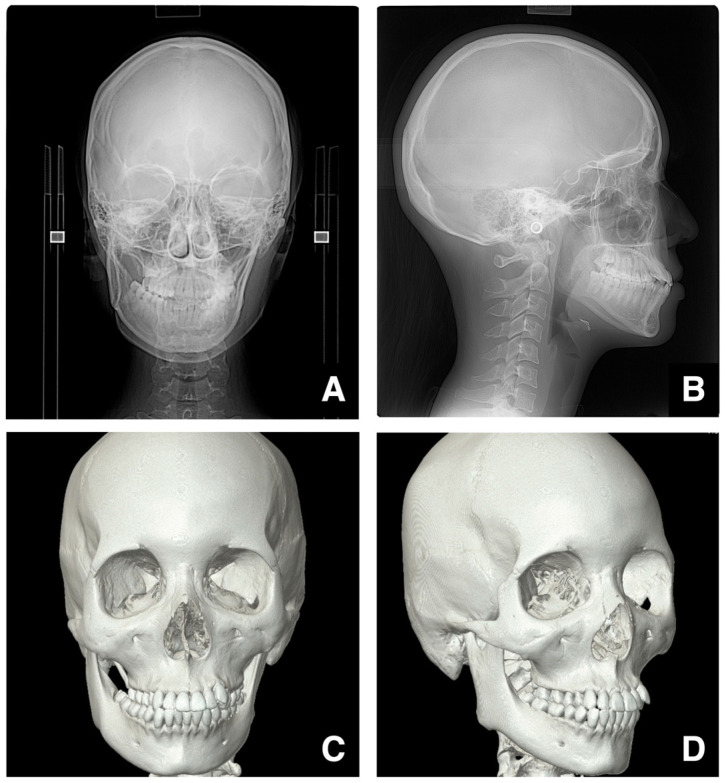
Three-dimensional reconstructed images using CT data and cephalometric radiographs in skeletal mandibular prognathism with facial asymmetry. (A) Frontal cephalometric radiograph of a patient with skeletal mandibular prognathism and facial asymmetry. (B) Lateral cephalometric radiograph of the same patient. It is difficult to evaluate the right and left sides of the craniofacial skeleton separately. (C) Frontal view of a 3D reconstructed image created from MDCT image data (slice thickness, 0.5 mm) of the same patient. (D) Right oblique view of the same 3D reconstructed image. Permission has been obtained to publish all figures.
